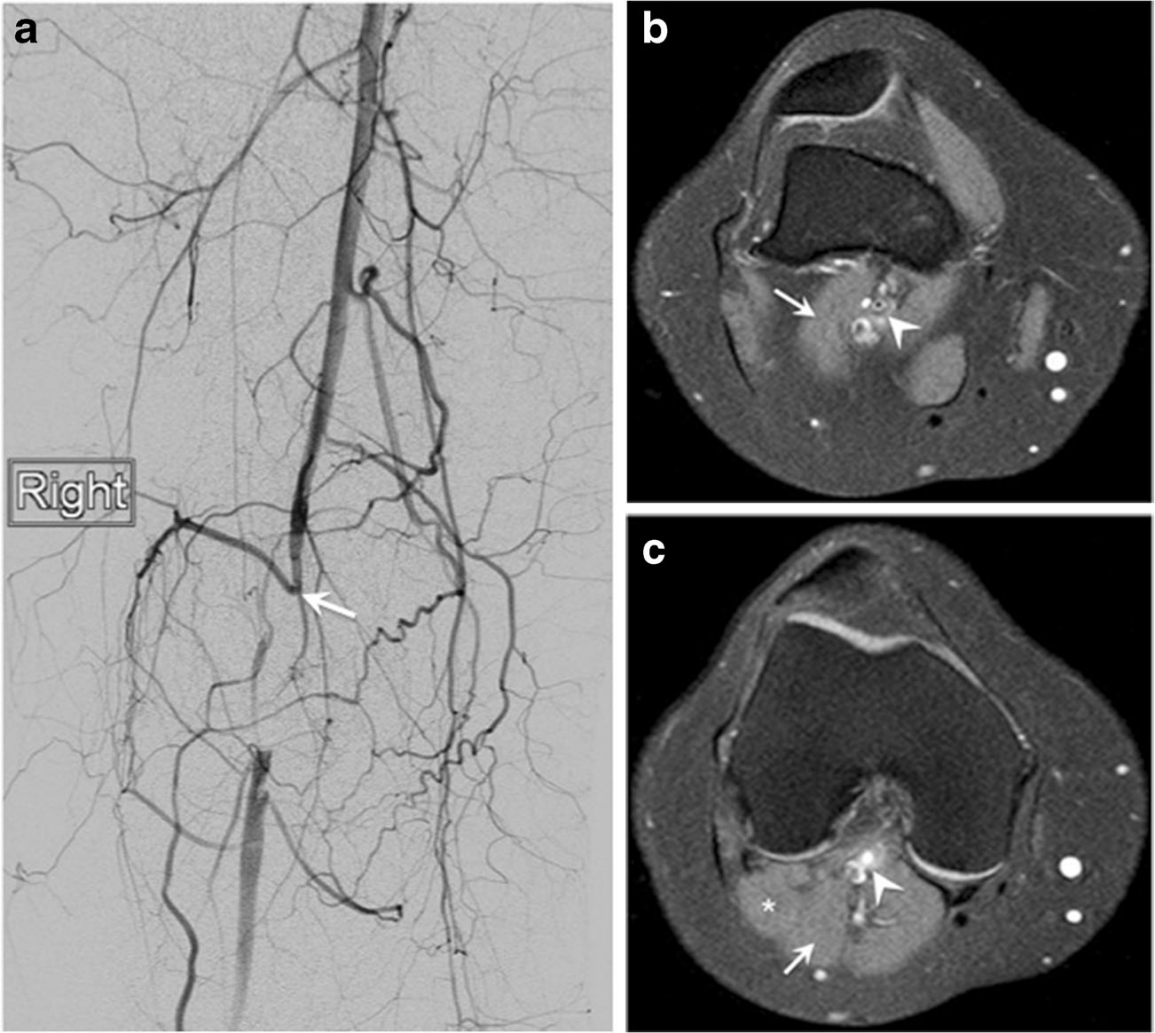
Popliteal artery entrapment syndrome (PAES) in a 31-year-old woman admitted to the hospital because of a 6-week history of right calf pain and numbness. Patient also reported half-block claudication. (a) Lower extremity digital subtraction angiography reveals obliteration of the popliteal artery (*arrow*). Given the lack of risk factors for an arterial thrombus, an anatomic etiology was suspected. (b, c) Axial selective partial inversion recovery (SPIR) MR images of the right knee showing an accessory slip of the lateral head of the gastrocnemius muscle (*arrow*) crossing anterolateral to the popliteal vessels, with resulting compression of the popliteal artery (*arrowhead*). The accessory slip (*arrow*) joins the lateral head of the gastrocnemius distally (*star*). This finding supports a diagnosis of PAES
